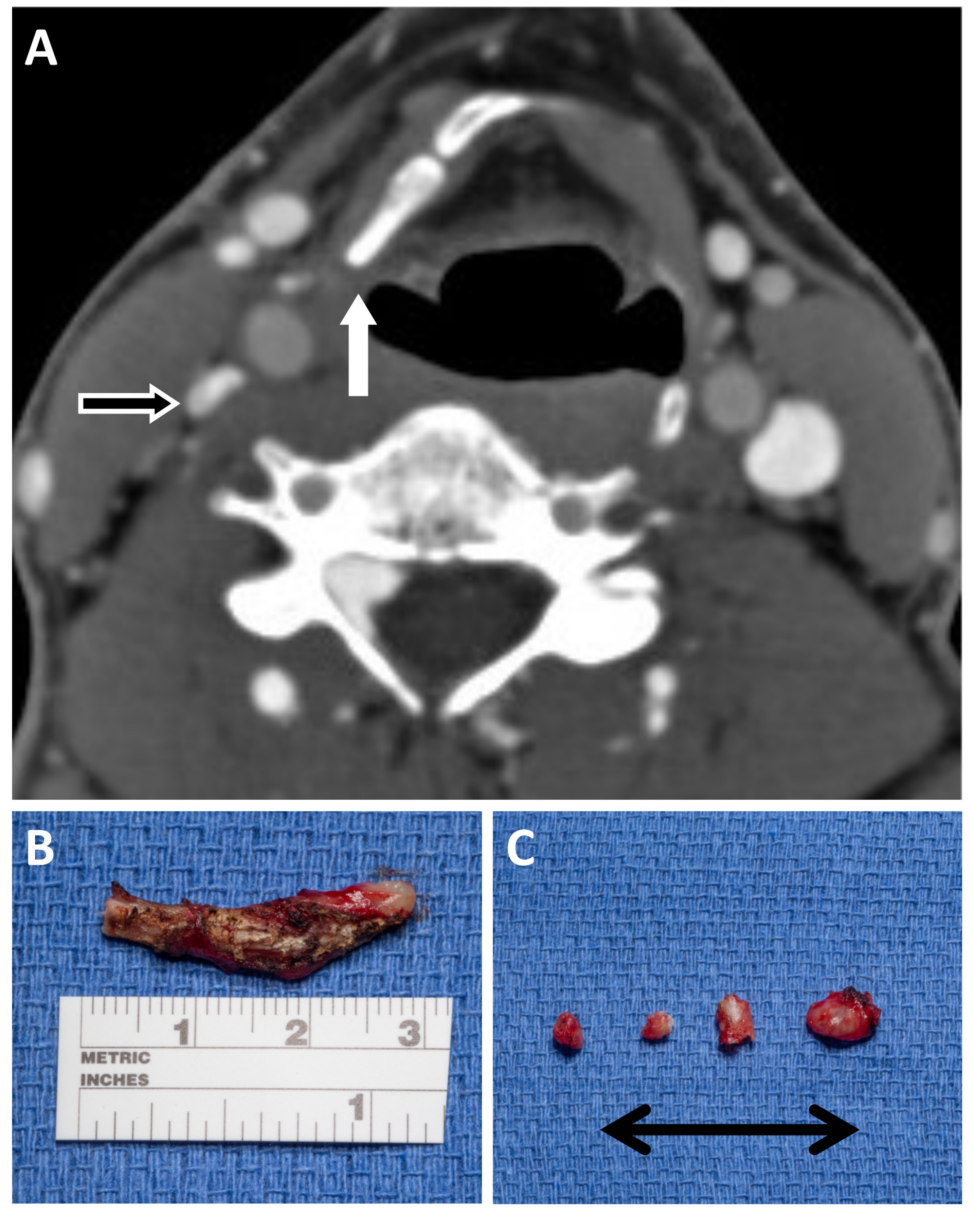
Postoperative venogram and intraoperative pathology. Postoperative axial CTV image showing resection of the hypertrophied segment of the hyoid bone (white arrow) and decompression of the right IJV (black and white arrow). (B, C) Intraoperative images showing resected sections of the (B) hyoid bone measuring nearly 3 cm and (C) fragments of the resected thyroid cartilage. CTV, computed tomography venogram; IJV, internal jugular vein
